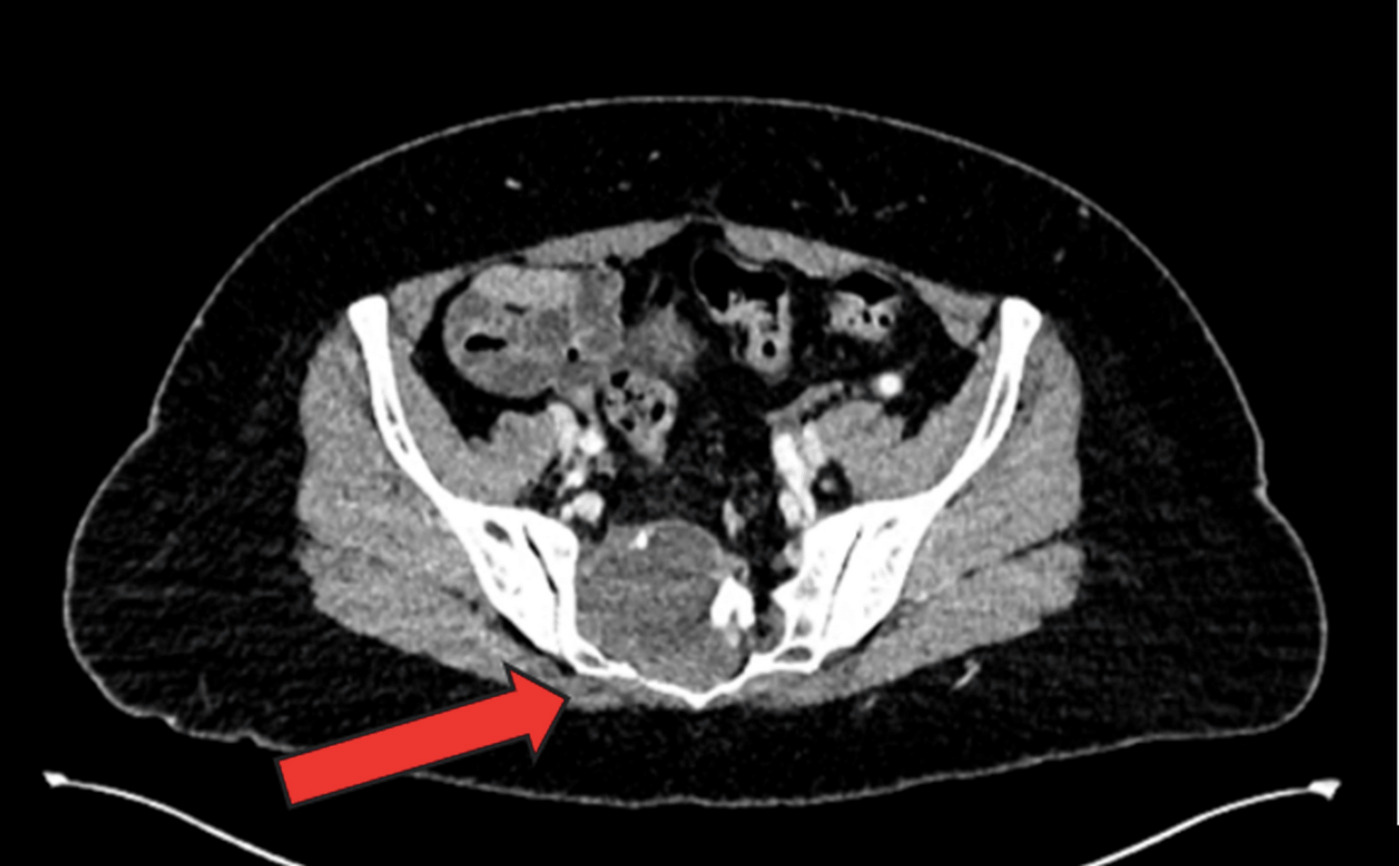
CT abdomen and pelvis (CT AP) demonstrating a right paracentral sacral mass with extra-osseous intra-abdominal extension, measuring approximately 5.5 × 3.6 cm, with mixed enhancing and non-enhancing areas and likely nerve root impingement (demarcated by red arrow).
